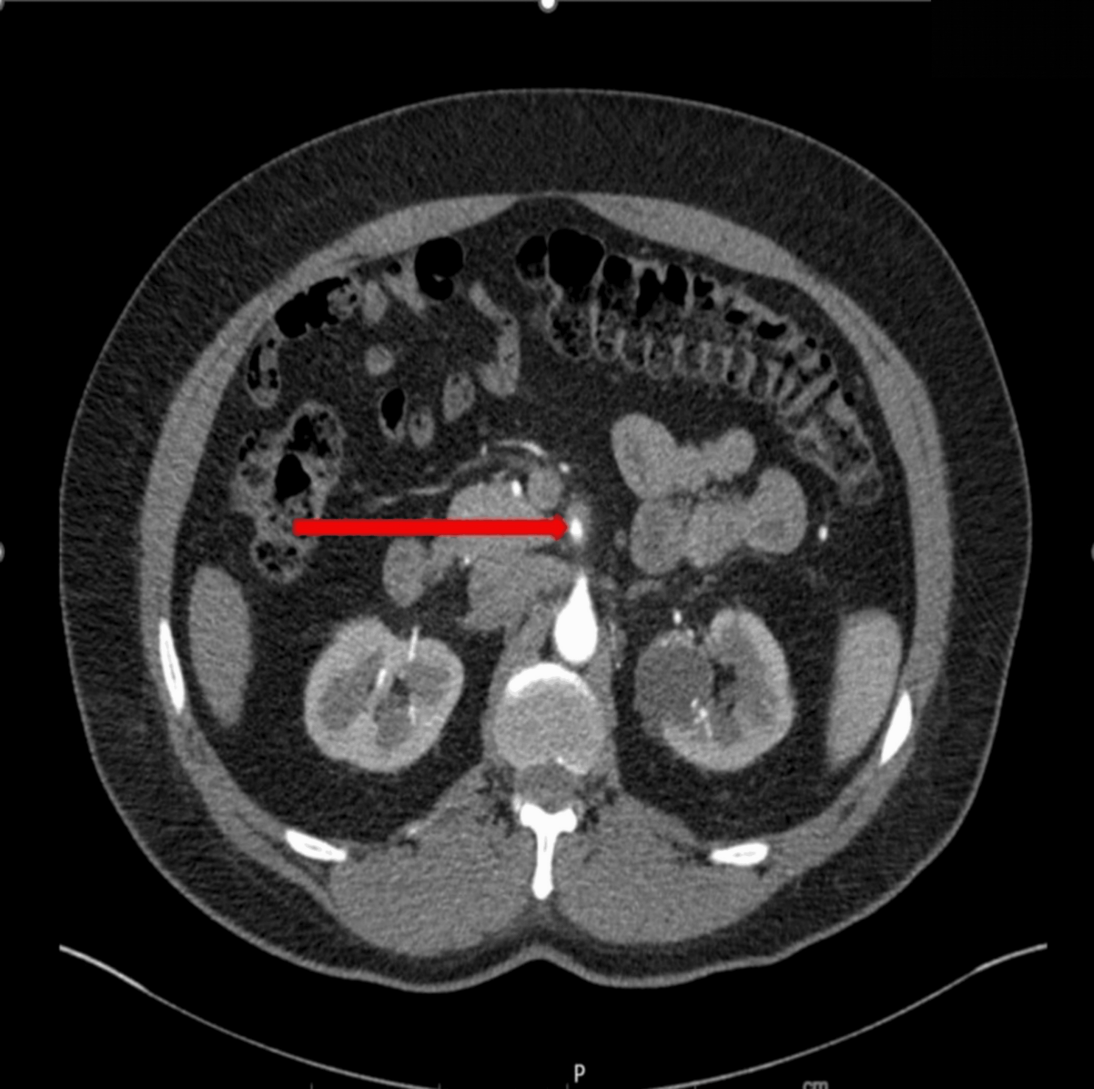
CT angiography of the abdomen and pelvis (axial section) showing superior mesenteric artery dissection with intramural hematoma.
